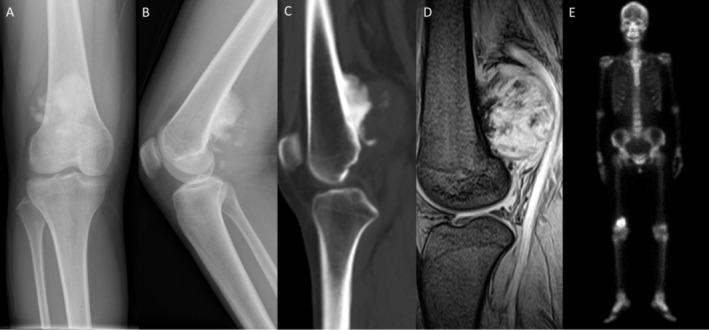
Preoperative anteroposterior (A) and lateral (B) X‐ray, CT (C), MRI (D), and SPECT scan (E) of a 30‐year‐old patient with highly differentiated and low‐grade parosteal osteosarcoma involving the right femur.
